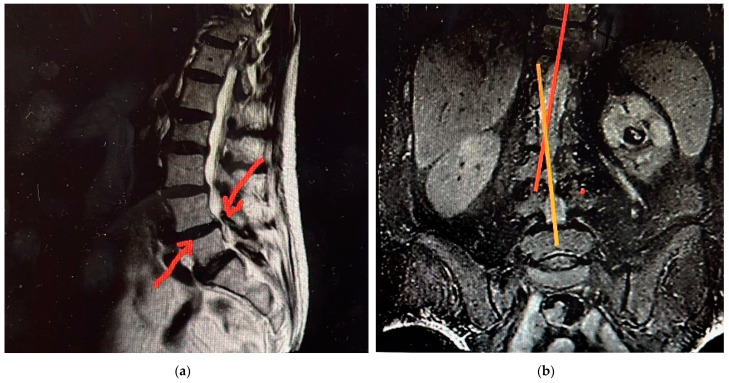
(a,b) MRI images of the spine for Case 1 following three weeks of sciatica and one week before the bilateral fracture. The inflammation and the discal prolapse associated with lumbar scoliosis remarked could have led to instant pain and caused the fall. The femoral heads are not shown in this image.
